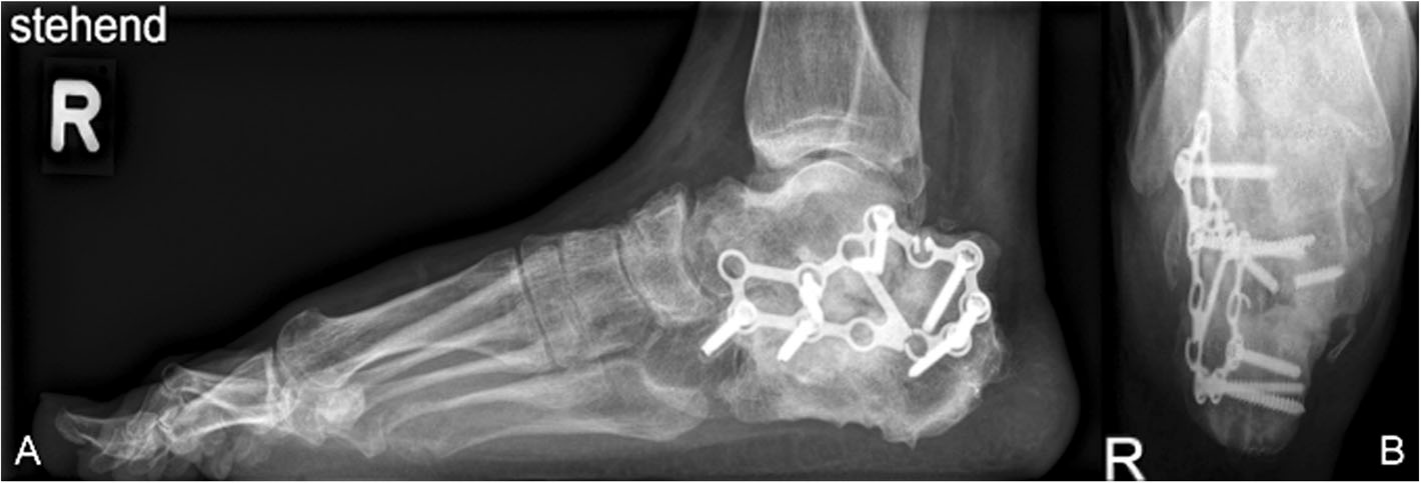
Case 2 Radiographs at follow-up Weightbearing right foot lateral (A) and calcaneus axial (B) 27 months post-surgery, AOFAS: 53
